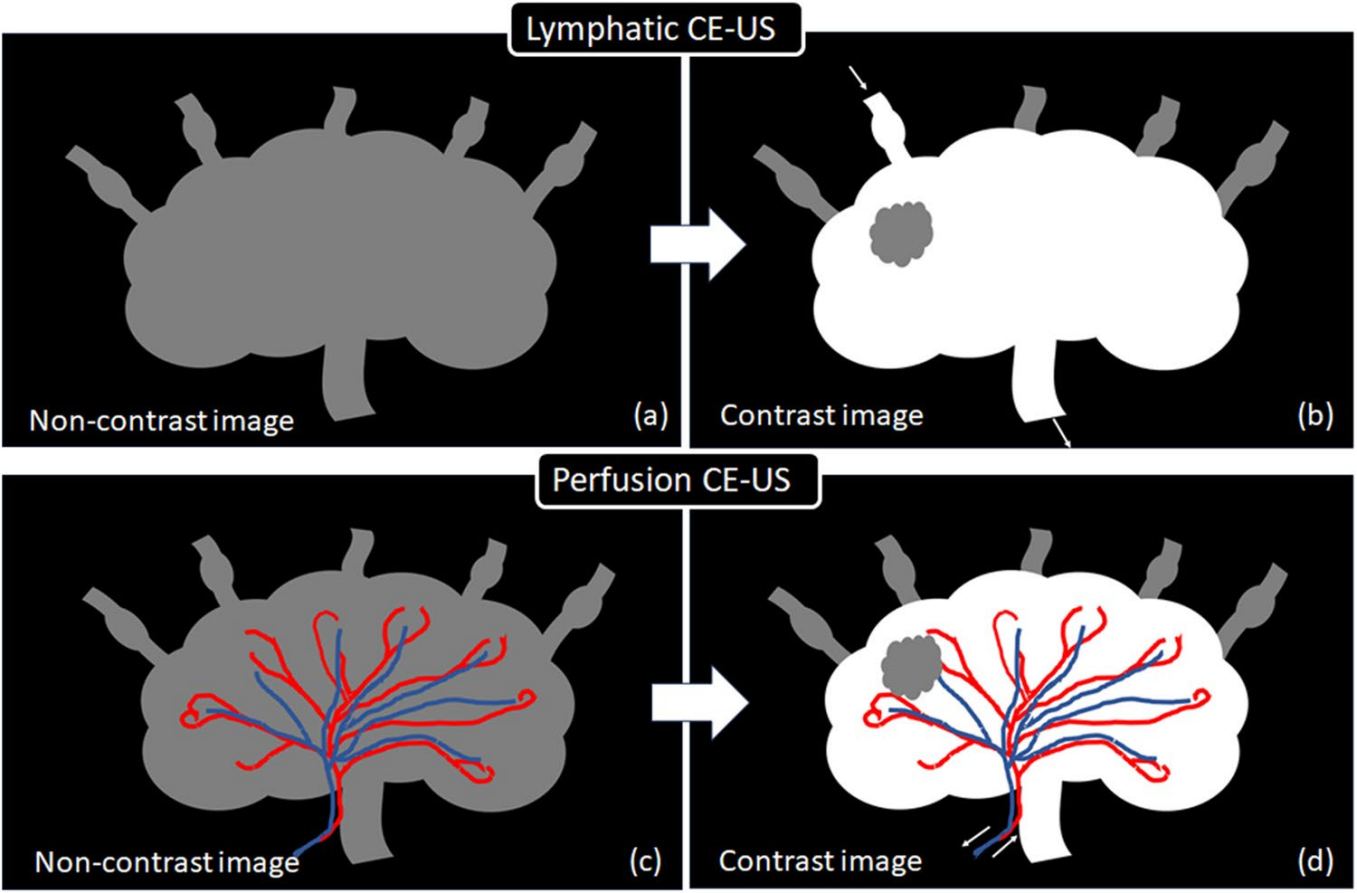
Mechanisms of detection of lymph node (LN) metastasis using lymphatic contrast-enhanced ultrasound (CE-US) and perfusion CE-US. In lymphatic CE-US, the contrast agent flows into the LN from the afferent lymphatic vessel on the capsule side, fills the LN, and is excreted from the efferent lymphatic vessel on the lymphatic hilum (b). Compared to the non-contrast image (a), the internal structures of normal LNs are contrast-enhanced, while the metastatic areas are not contrast-enhanced and become defects. In perfusion CE-US, the contrast agent enters the LN through the artery of the lymphatic hilum, perfuses the LN, and drains out through the vein of the lymphatic hilum. Compared to the non-contrast image (c), the internal structures of normal LNs are contrast-enhanced, while metastatic areas are not contrast-enhanced, resulting in a perfusion defect
